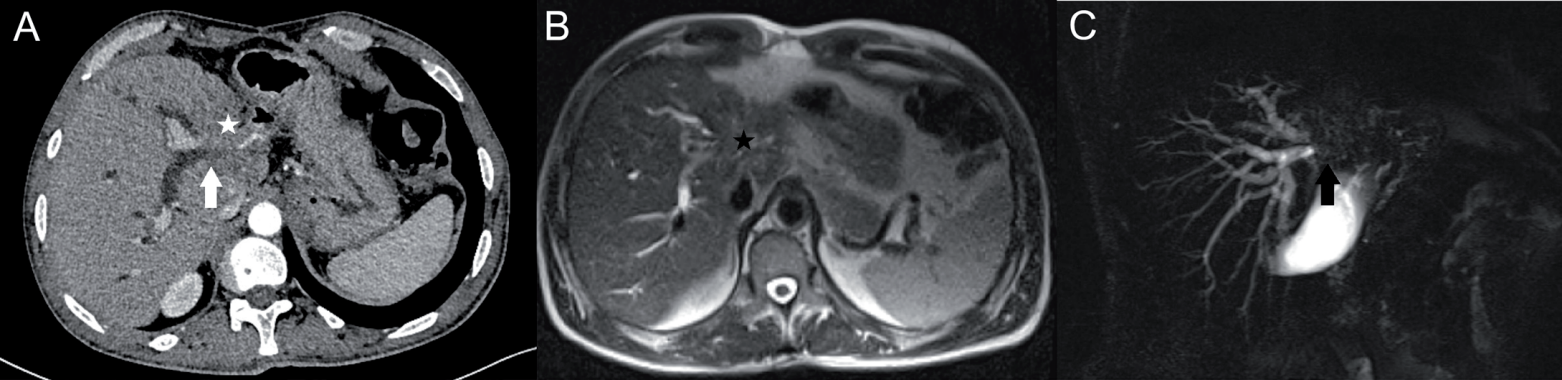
Axial contrast-enhanced computed tomography (CT) image (A), axial T2-weighted magnetic resonance image (B). and thick slap MRCP (Magnetic resonance cholangiopancreatography) image (C) show an alveolar echinococcosis lesion with Klatskin tumor-like involvement is observed in the left lobe of the liver (white and black stars in A and B). Note that the intrahepatic biliary tract is interrupted at the bifurcation level (white and black arrows in A and C), and capsular retraction is observed in the left lobe of the liver adjacent to the lesion.
